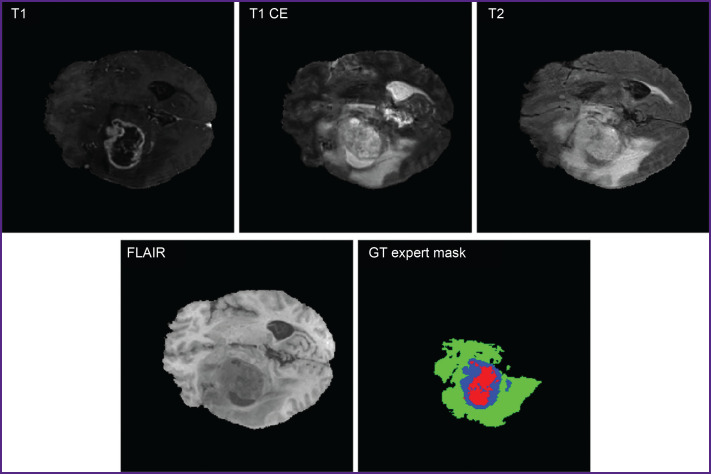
Brain MR image in different modalities (T1, T1 CE, T2, FLAIR) and color segmentation (GT expert mask) of three classes: necrotic and non-enhancing tumor core (NCR/NET — red), peritumoral edema (ED — green) and enhancing tumor (ET — blue)
